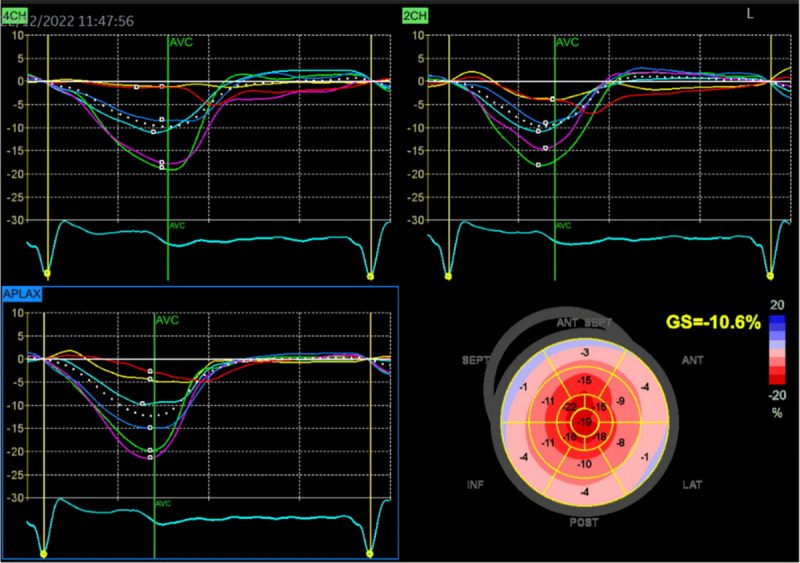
Two-dimensional speckle-tracking strain imaging echocardiography of a patient with ATTRwt-CM (the same patient as in Figure 2). Reduced left ventricular longitudinal strain in the middle and basal segments with relatively preserved strain in the apex (i.e., apical sparing) is observed. A bull’s eye is shown in the lower right panel (Images courtesy of Centro Hospitalar Universitário Lisboa Norte, Lisboa, Portugal).
